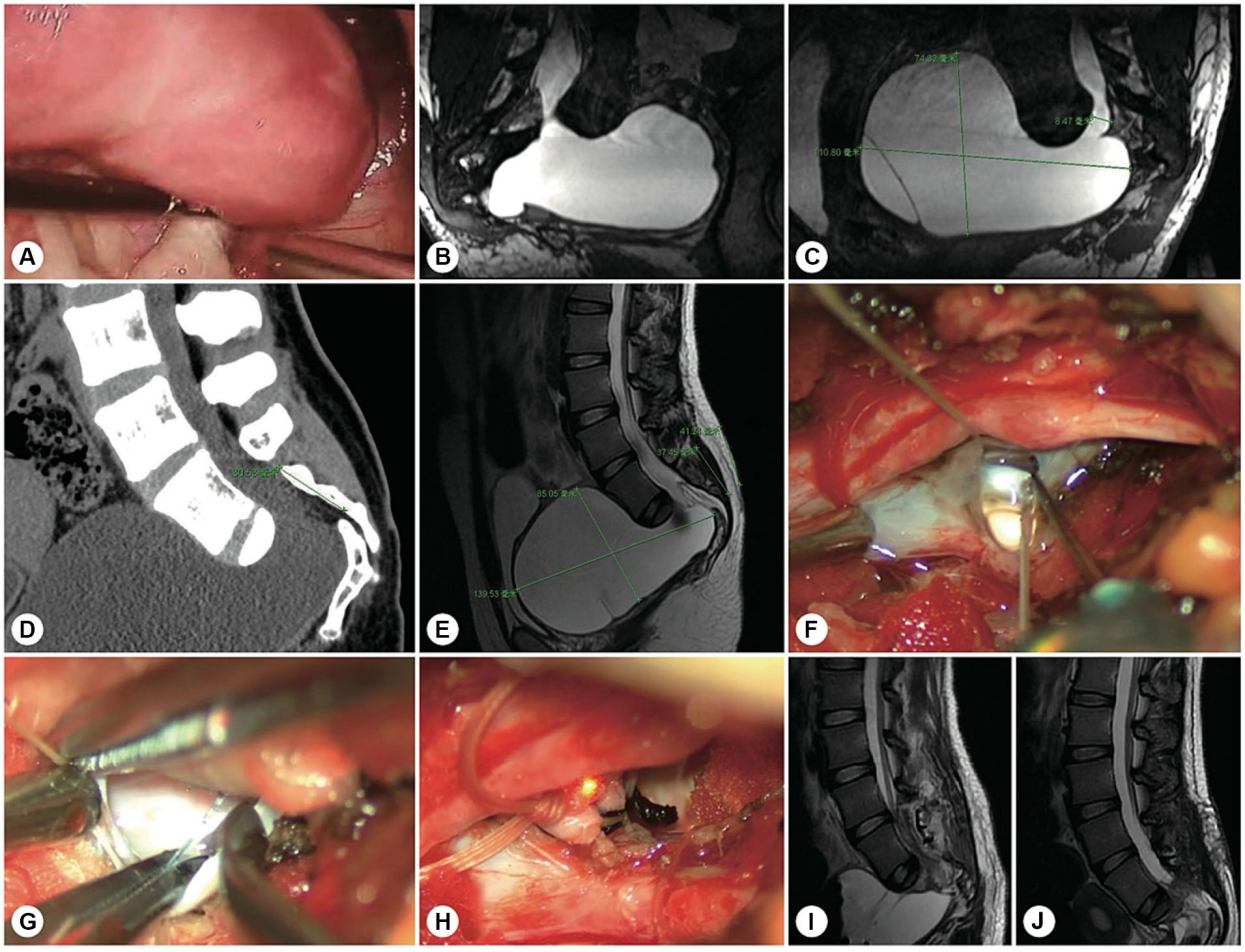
A representative patient with both sacroanterior and sacral cysts. A 22 years-old woman presenting with abdominal pain was found to have a pelvic mass. (A) Laparoscopic exploration revealed that the pelvic lesion was connected to the sacral canal. (B) Coronal MR image showing wide neck leakage between the end of the dural sac and the sacral canal. (C) Sagittal MR image revealing a giant sacroanterior cyst connected to a sacral cyst without nerve root fibres inside. (D) Sagittal CT image showing a sacroanterior bone defect. (E) A precise incision was made. (F) Intraoperatively, abundant cerebrospinal fluid leaked from the orificium, suggesting high intracystic pressure. (G) No spinal nerve root fibres were identified in the leakage orificium. (H) The leakage orificium was ligated. (I) On MRI, 1 week after the operation, the sacroanterior cyst had shrunk. (J) Three months after surgery, the sacroanterior cyst had almost completely disappeared on MRI.
